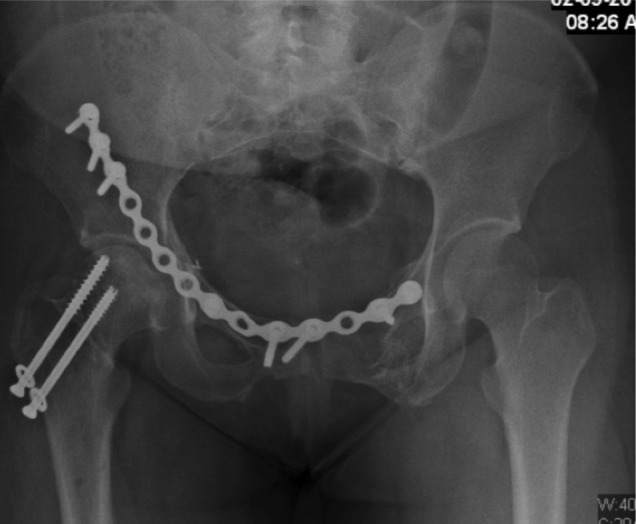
Pelvic radiograph following open reduction and ring internal fixation showing acceptable alignment, screw fixation neck of femur in situ.
